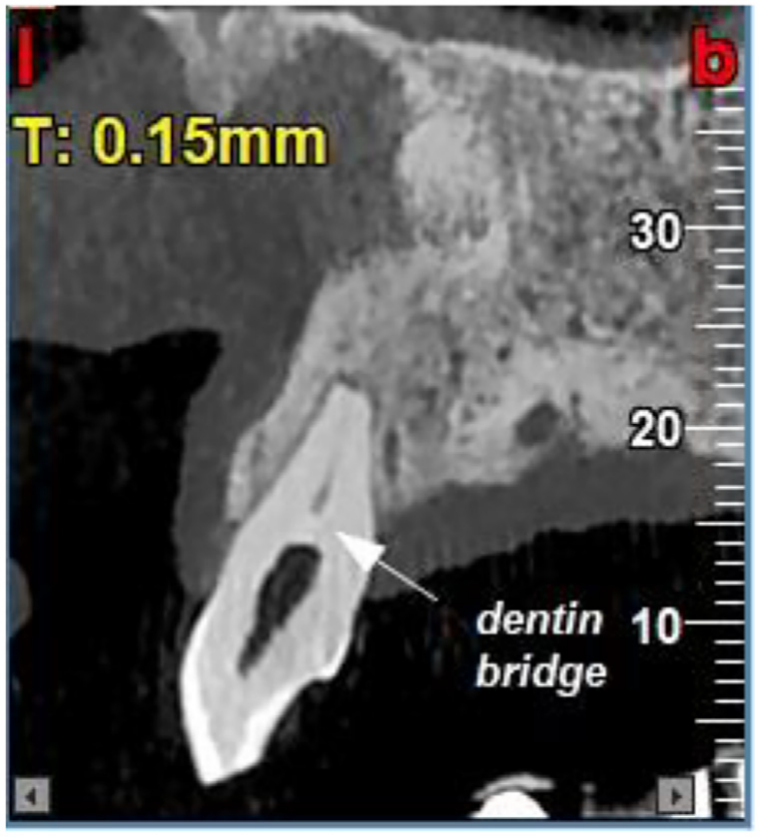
Sagittal view of CBCT image shows dentin bridge beneath the area of internal root resorption.
